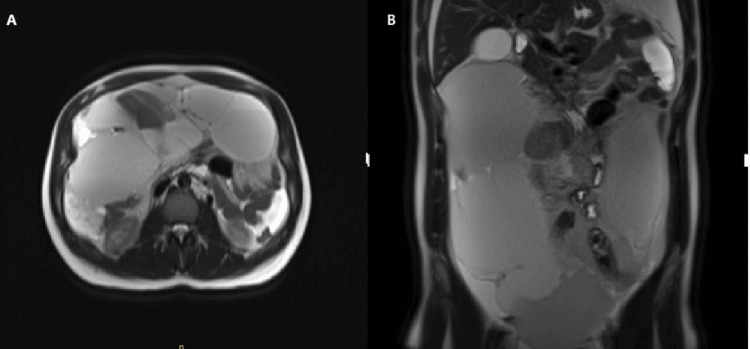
An MRI transverse (A) and coronal (B) sections of the abdomen showed a multiloculated cystic lesion measuring approximately 9.0 x 21.3 x 26 cm
